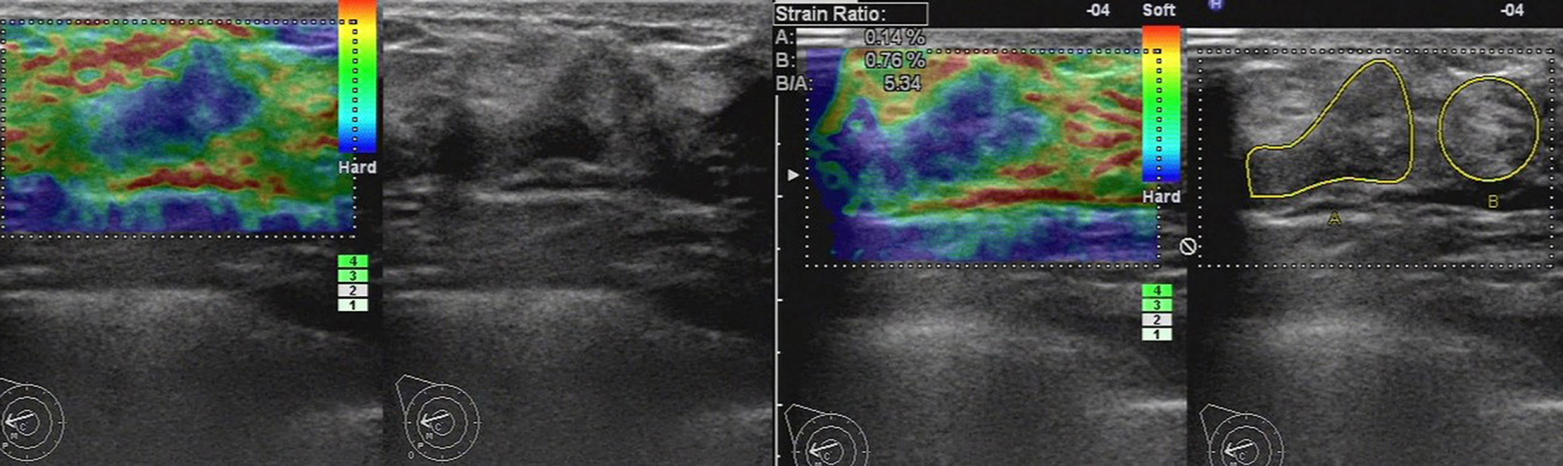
Ultrasonic elastographic images of a IDC in a 41-year-old woman. Left: The lesion was scored 3–4 with the 5-point scoring method. Right: The strain ratio was 5.34(malignant) with the strain ratio method at the same breast tissue depth as the reference.
